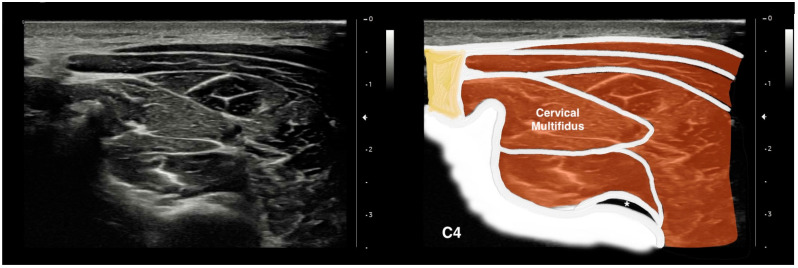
Ultrasound imaging of the cervical multifidus muscle at the C4 level. *: C4/C5 zygapophyseal joint; yellow: Nuchal ligament.
